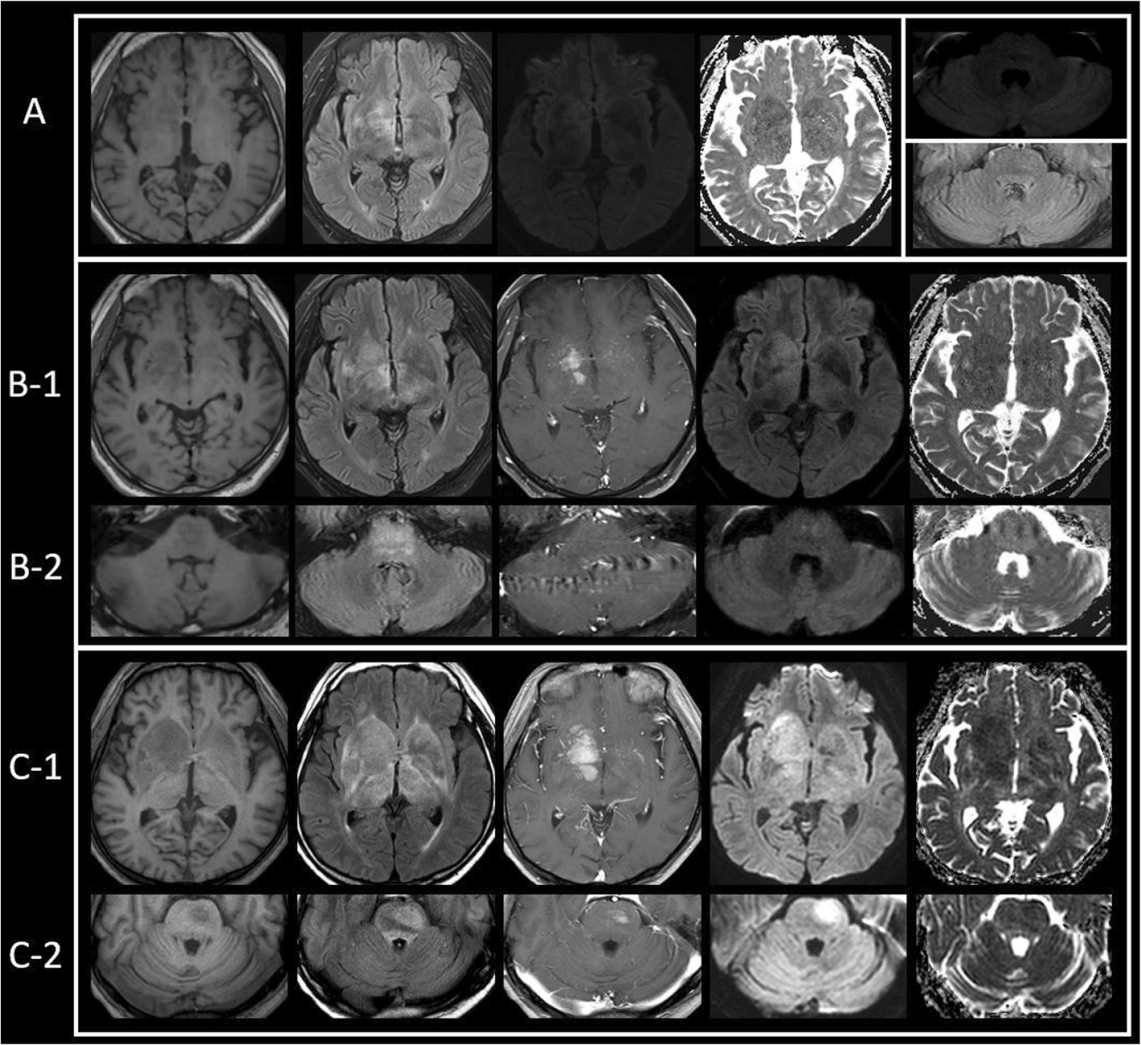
Serial brain magnetic resonance imaging (MRI) of the patient. (A) First MRI after the onset of symptoms. The right-sided basal ganglia had a mild, low signal intensity on the T1-weighted image and high signal intensity on the fluid attenuated inversion recovery (FLAIR) image. However, matching of diffusion weighted image (DWI) and apparent diffusion coefficient (ADC) was not clearly observed. The pons and cerebellar pontine angle don’t have any abnormal signal intensity on the DWI and FLAIR image. Abnormal signal intensity was not observed in FLAIR in both medial temporal lobes. (B) Follow-up MRI after symptom aggravation. Compared with first MRI, the signal intensity in the T1-weighted image was pronounced and the size of the high signal intensity on the FLAIR image increased. Furthermore, enhancement of the lesion was observed as well as matching of the DWI and ADC. (B-1) A new lesion, which was not seen in the first MRI, was observed in the pons. Low signal intensity was observed on the T1-weighted image and high signal intensity on the FLAIR image. Matching of the DWI and ADC was observed. (B-2). (C) After plasmapheresis, the patient’s condition worsened, and follow-up brain MRI was performed. The basal ganglia enhancement was increased and the edema worsened. The matching of DWI and ADC became more prominent. (C-1) Enhancement lesion was observed in the pons. (C-2)
